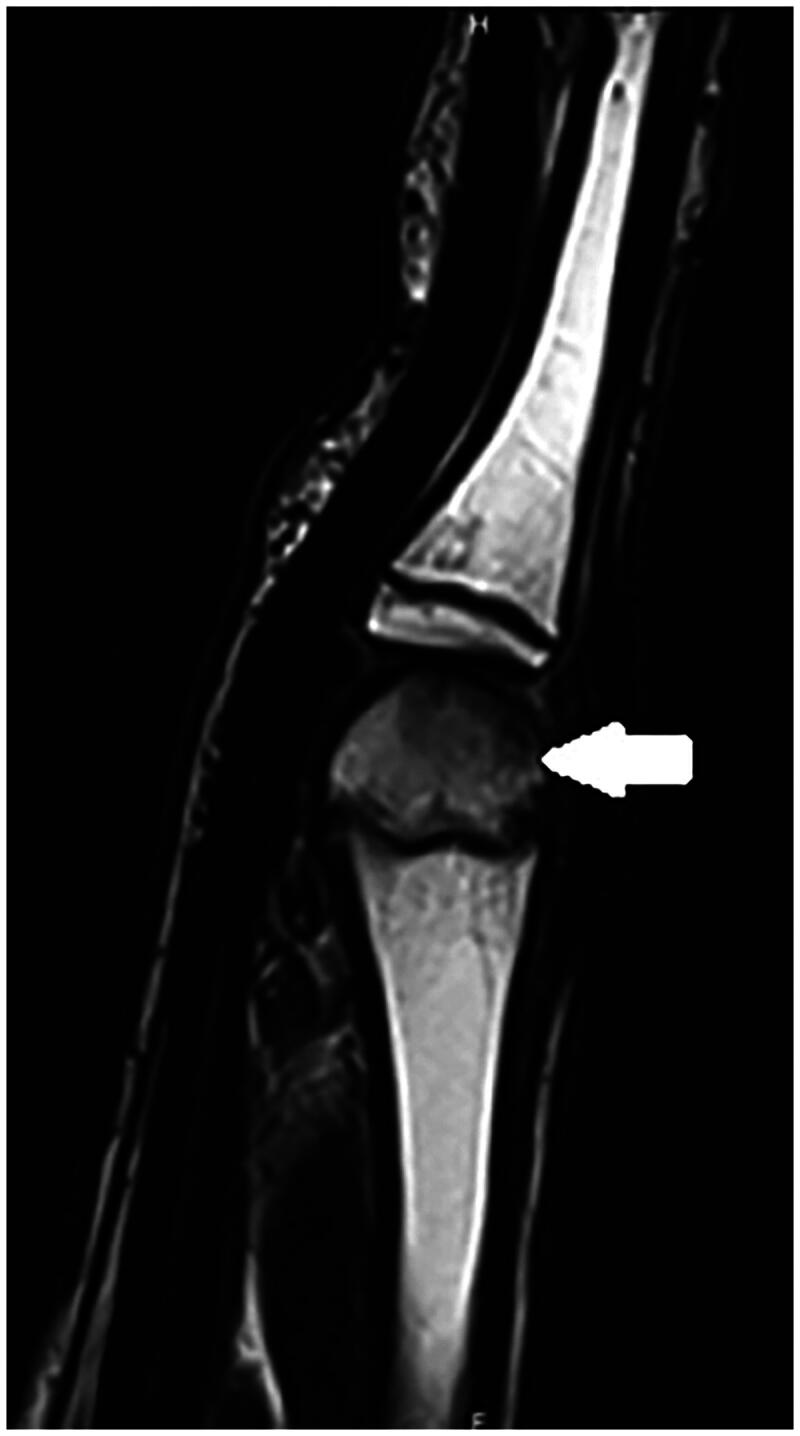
First MRI, July 2022. Subchondral fracture of the distal epiphysis of the third metacarpal head, with probable avascular necrosis and preserved viability in T1 weighted images.
